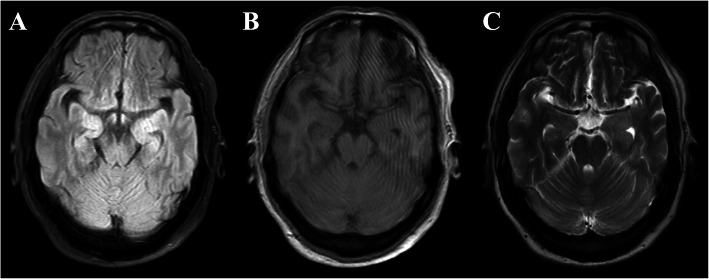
Magnetic resonance imaging of the patient. T2-weighted fluid-attenuated inversion recovery (FLAIR) showed slightly elevated signals within bilateral medial temporal lobes (a), whereas no abnormalities were seen on the T1 or T2 scans (b-c)
